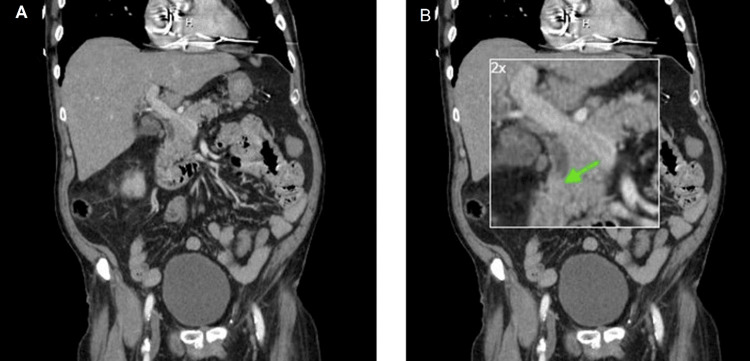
CT abdomen and pelvis (coronal) demonstrating (A) distal bile duct filling defect (green arrow) and (B) with 2x magnification
